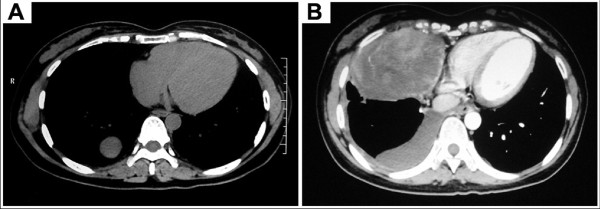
Recurrence of SFTP in a case. (A) CT scan showed a prevalent intrapulmonary growth (the so-called inverted fibroma). (B) Another smoothly marginated abnormal mass occurred at right thorax with pleural effusion 6 months after surgery.
